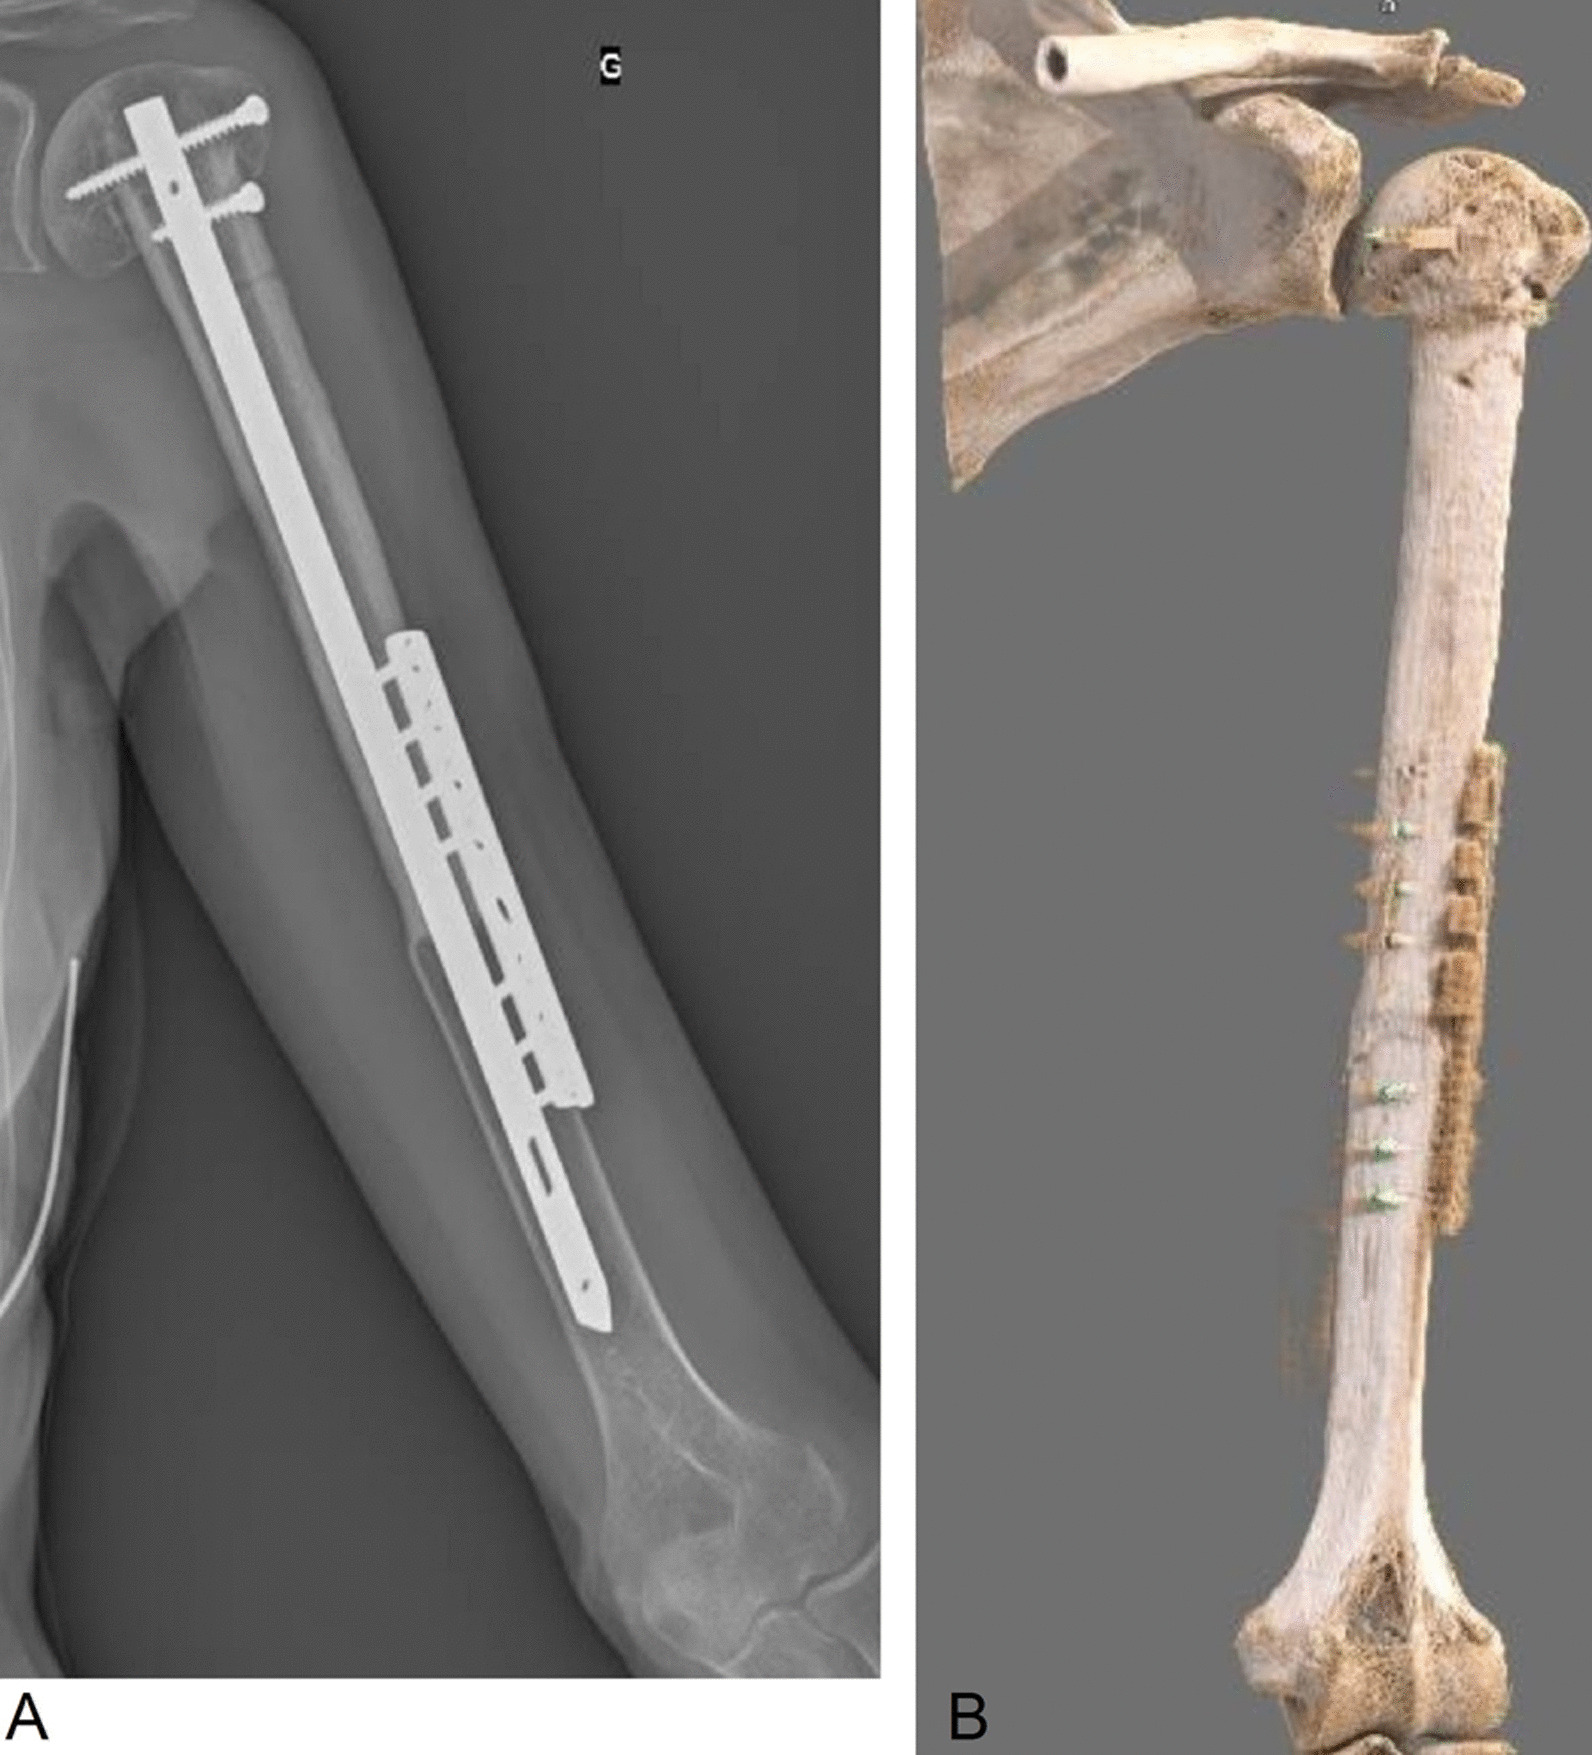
June 2020 examination with standard X-ray (A) and 3D-computerized tomography reconstruction (B)
